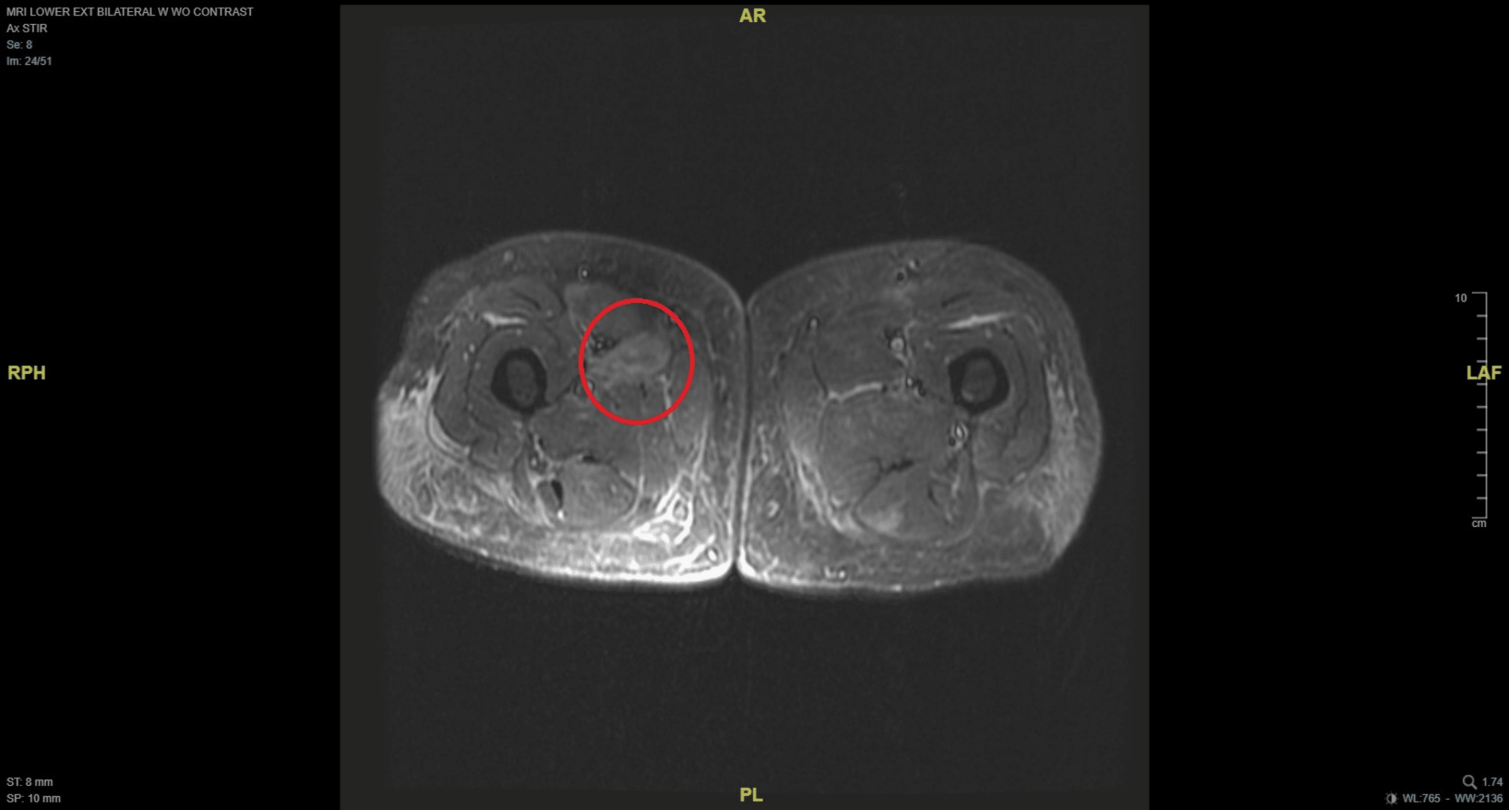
Axial MRI of both lower extremities with a red circle highlighting the area of hyperintensity in the right lower extremity.
